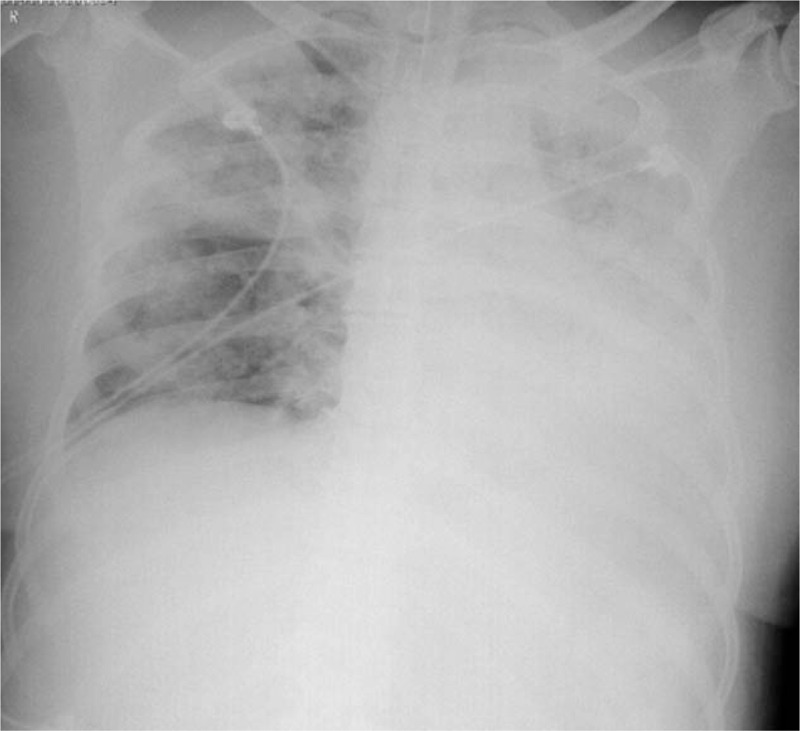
Chest X-ray of the female patient after 1 week of treatment revealed consolidations on the left lung and patchy shadow on the right lung. CT = computed tomography.
